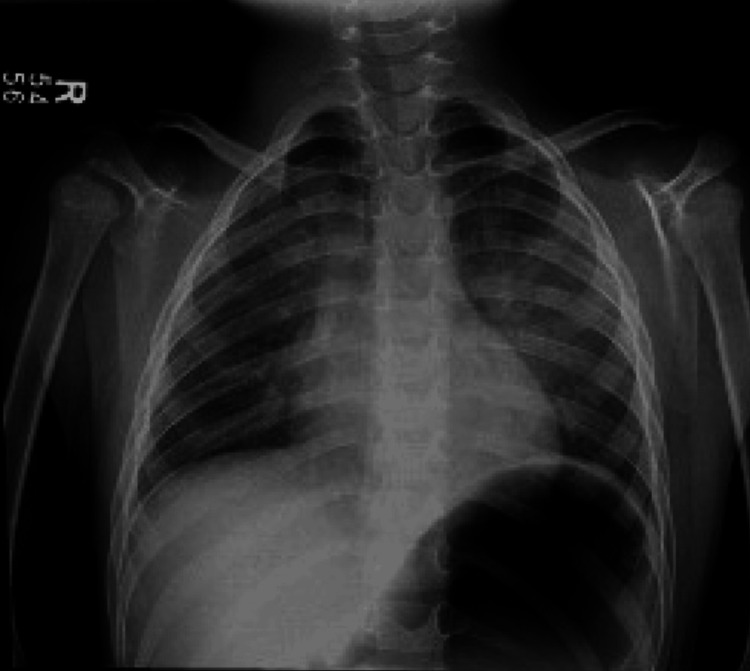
Postoperative chest X-ray showing clear lungs with no evidence of dental aspiration
